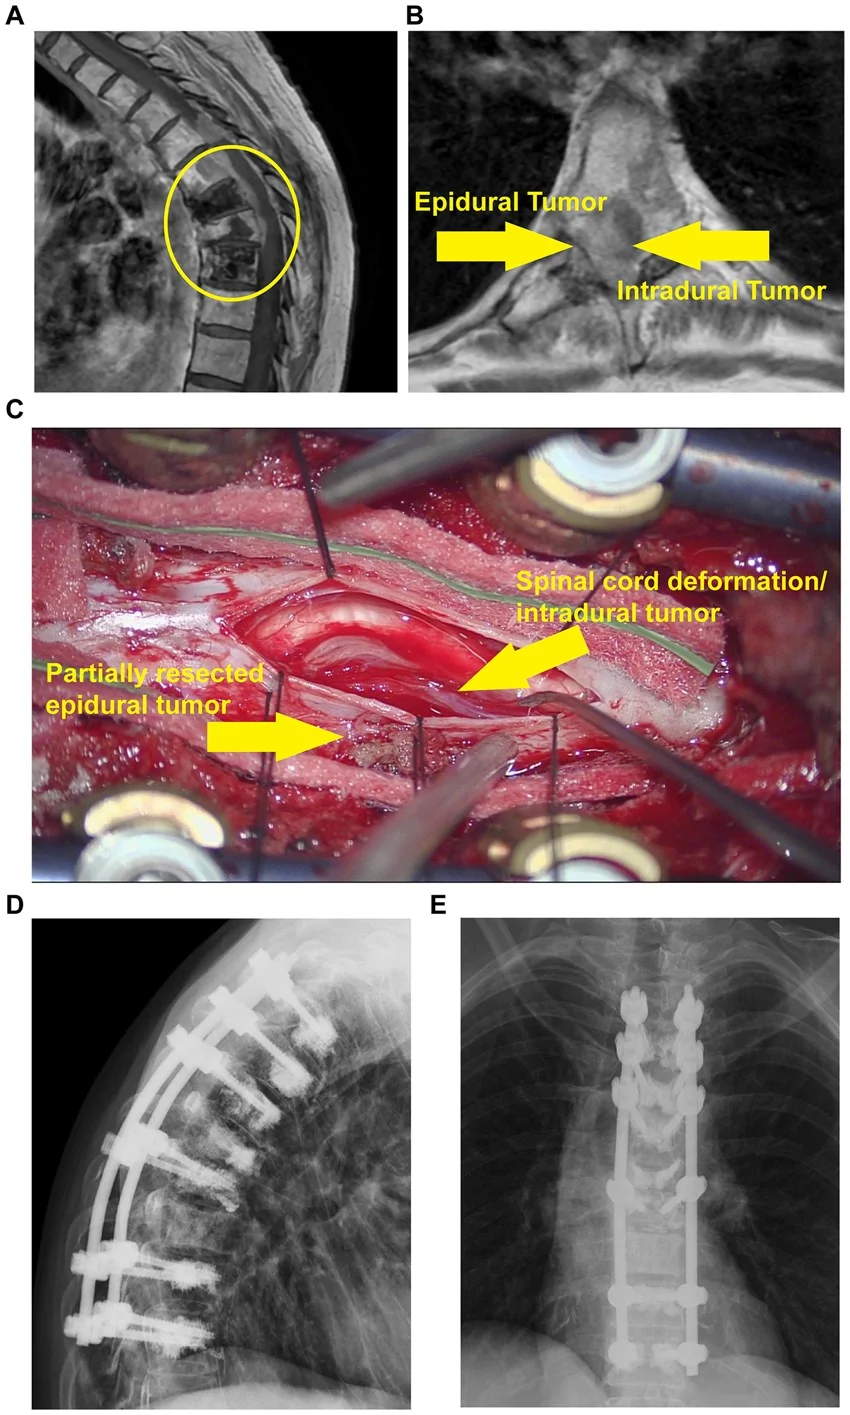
Clinical presentation of ER^+^/PR^+^ metastatic breast cancer to the spine. (A) Sagittal MRI with gadolinium contrast enhancement demonstrating the multilevel disease with multilevel fractures and circumferential epidural spinal cord compression. (B) Axial MRI with gadolinium contrast enhancement demonstrating intradural and epidural disease resulting in severe spinal cord compression. (C) Intraoperative snapshot demonstrating residual epidural disease as well as intradural disease compressing the deformed spinal cord. (D) Lateral and (E) Anterior-posterior x-rays showing the postoperative hardware with cement augmentation.
